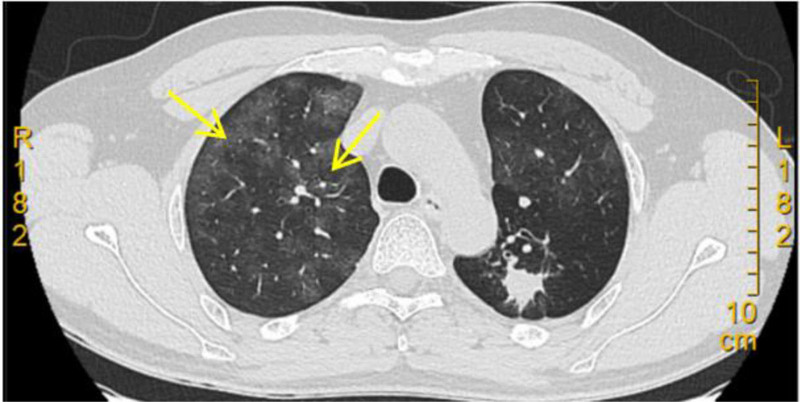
Chest CT scan on October 26, 2023. The lesions showed no significant changes (yellow arrow). CT = computed tomography.
